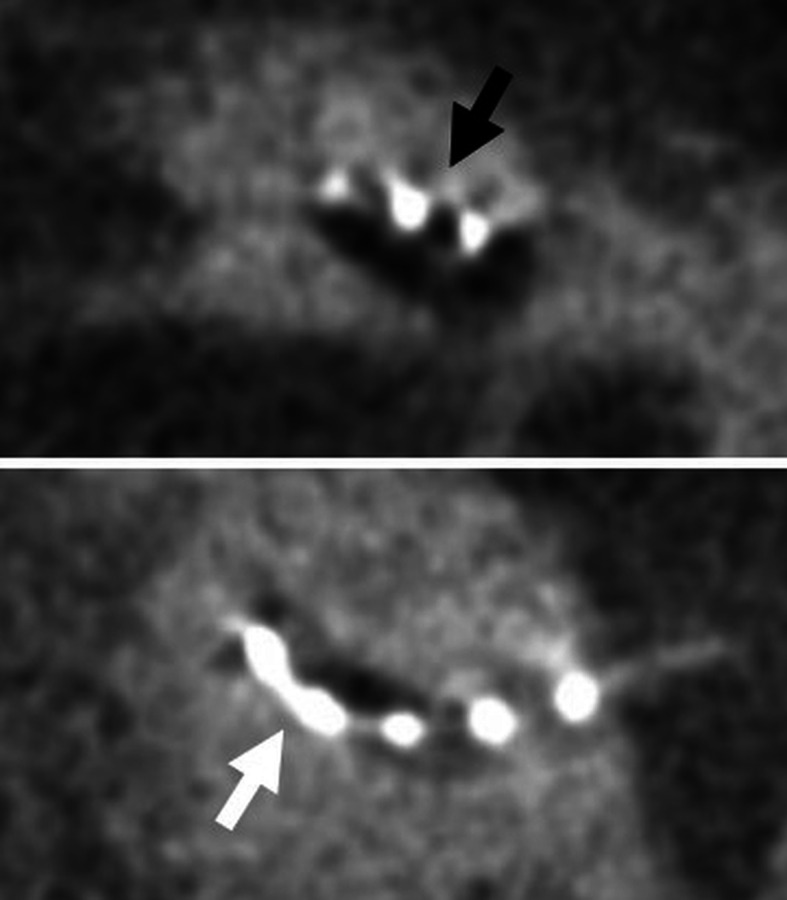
A 62-year-old male patient, with the scalar translocated electrode. CBCT axial images show translocation of the electrode array from scala tympani (white arrow) into scala vestibuli (black arrow). The electrode was inserted via cochleostomy
